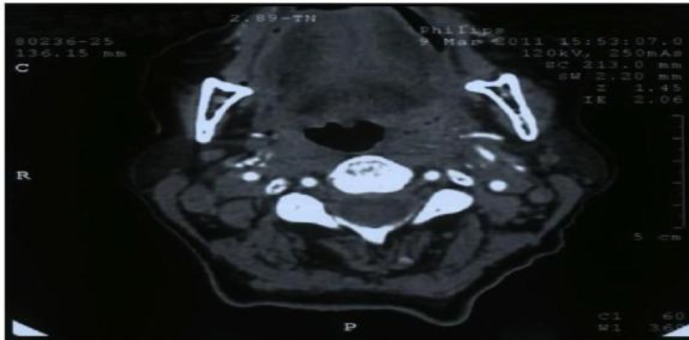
Axial computed tomography (CT) scan of neck showing an ill defined mass of hyp
